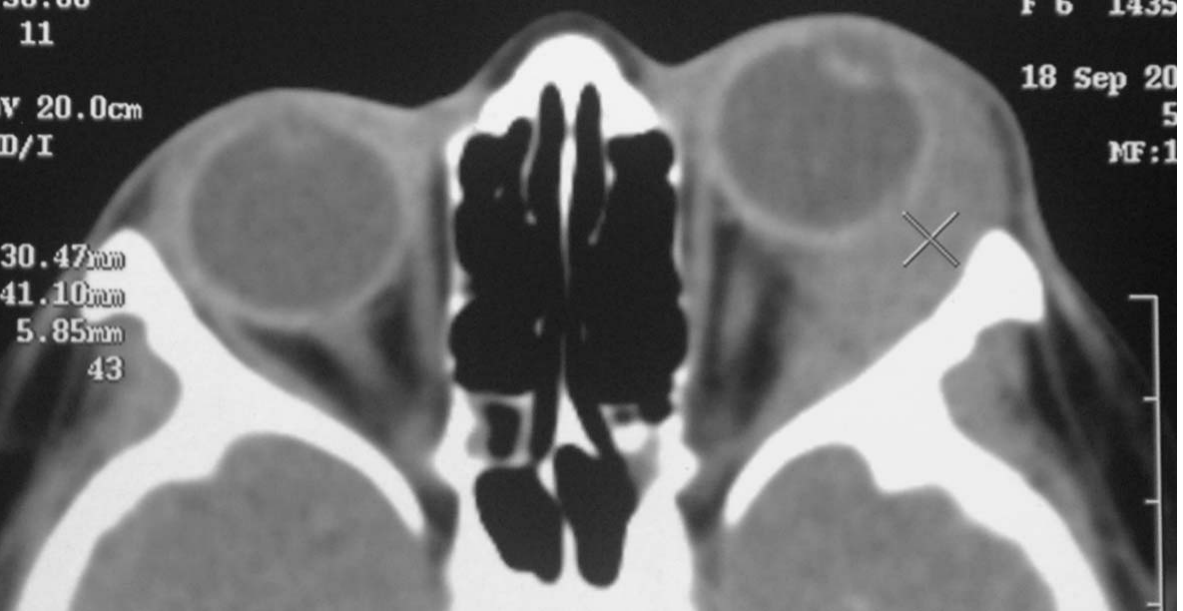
CT scan axial view revealed a diffuse soft tissue mass involving the left superotemporal orbit.
